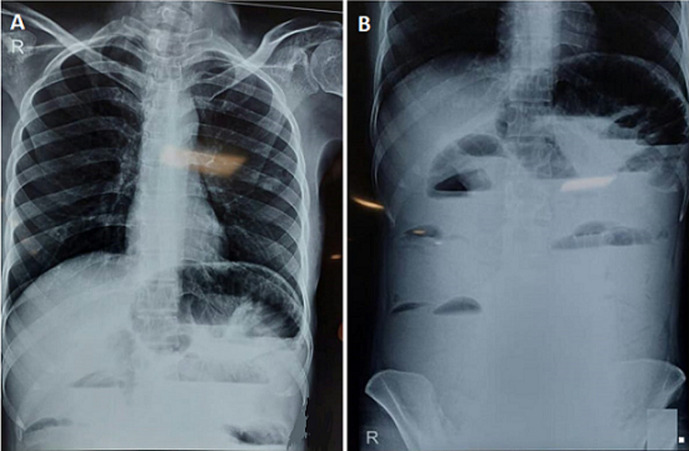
A) chest X-ray showing radiolucent gastric bubble over the left side; B) X-ray of abdomen showing multiple small bowel loops and one large bowel loop suggestive of obstruction distal to the transverse colon
